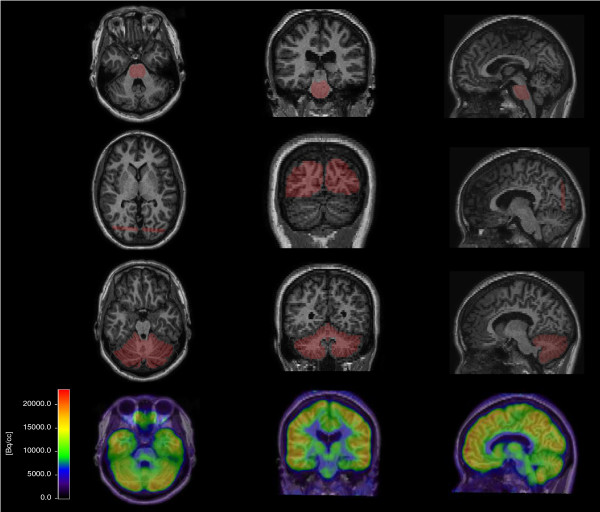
Co-registered T1-MR and FDG PET of a control. The ROI was defined on the co-registered T1-MR on sagittal, coronal, and axial slices. The upper row shows the ROI of the pons, the second row of the occipital lobe, and the third row of the cerebellum. For the occipital lobe, five slices were taken as the ROI from the coronal angle. The lower row shows the PET scan after T1-MRI fusion.
